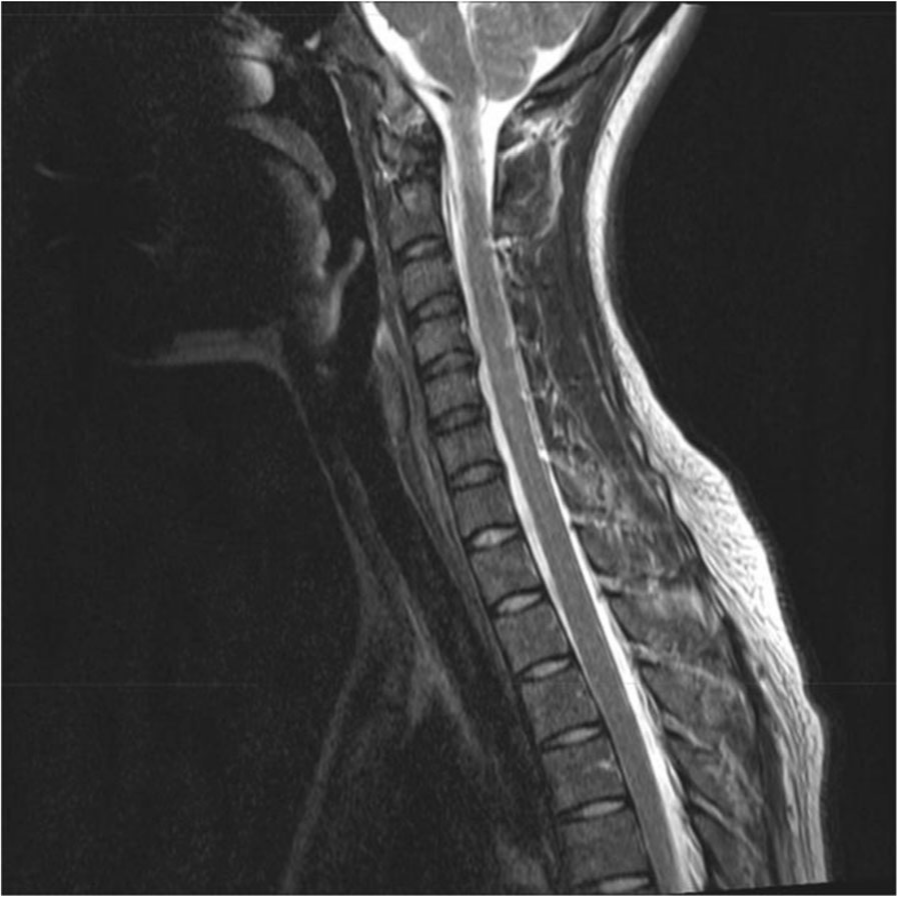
Cervical MRI of the patient
